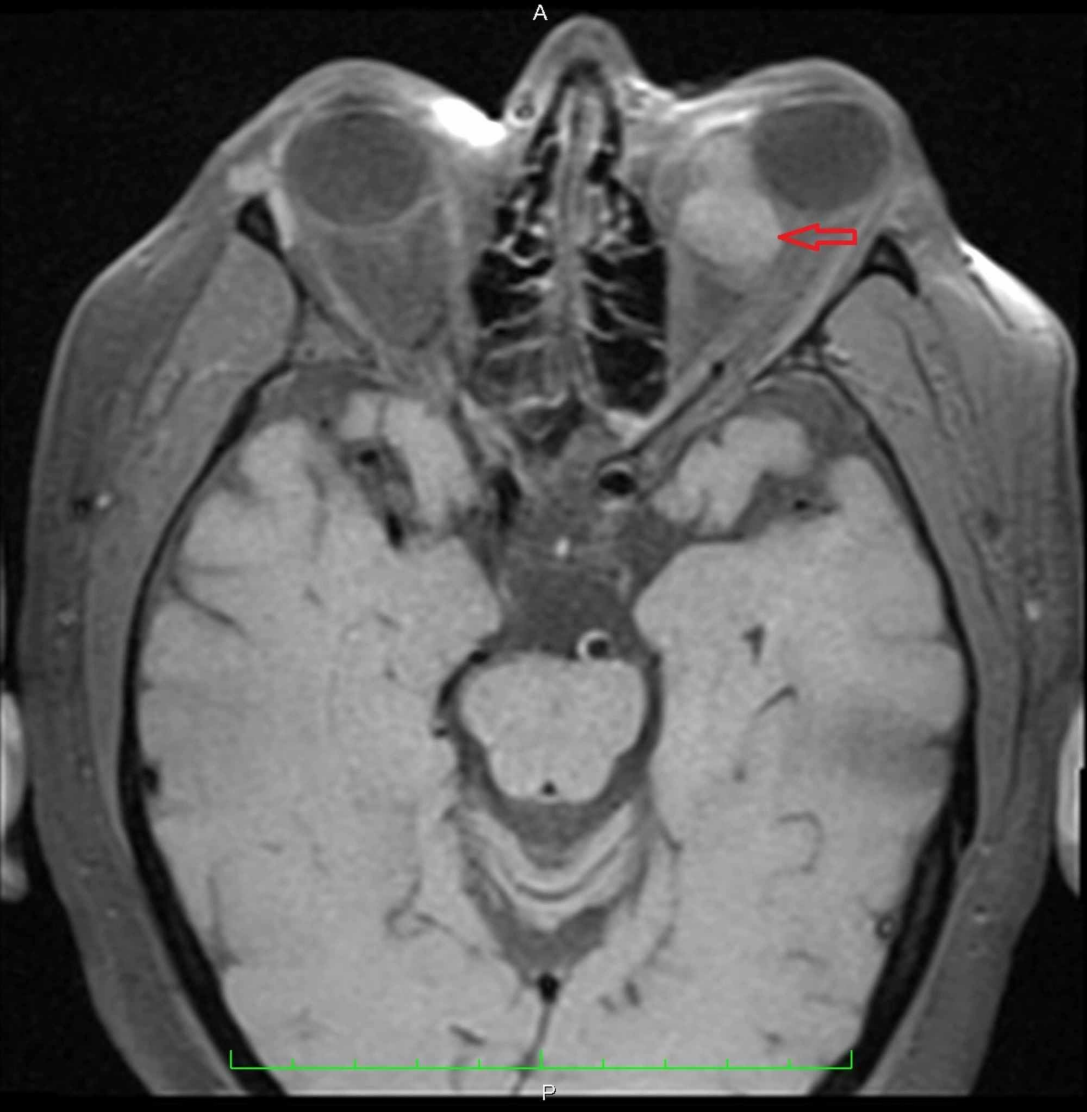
Transverse MRI of the orbits without contrast revealed a mass in the left orbit contributing to proptosis
